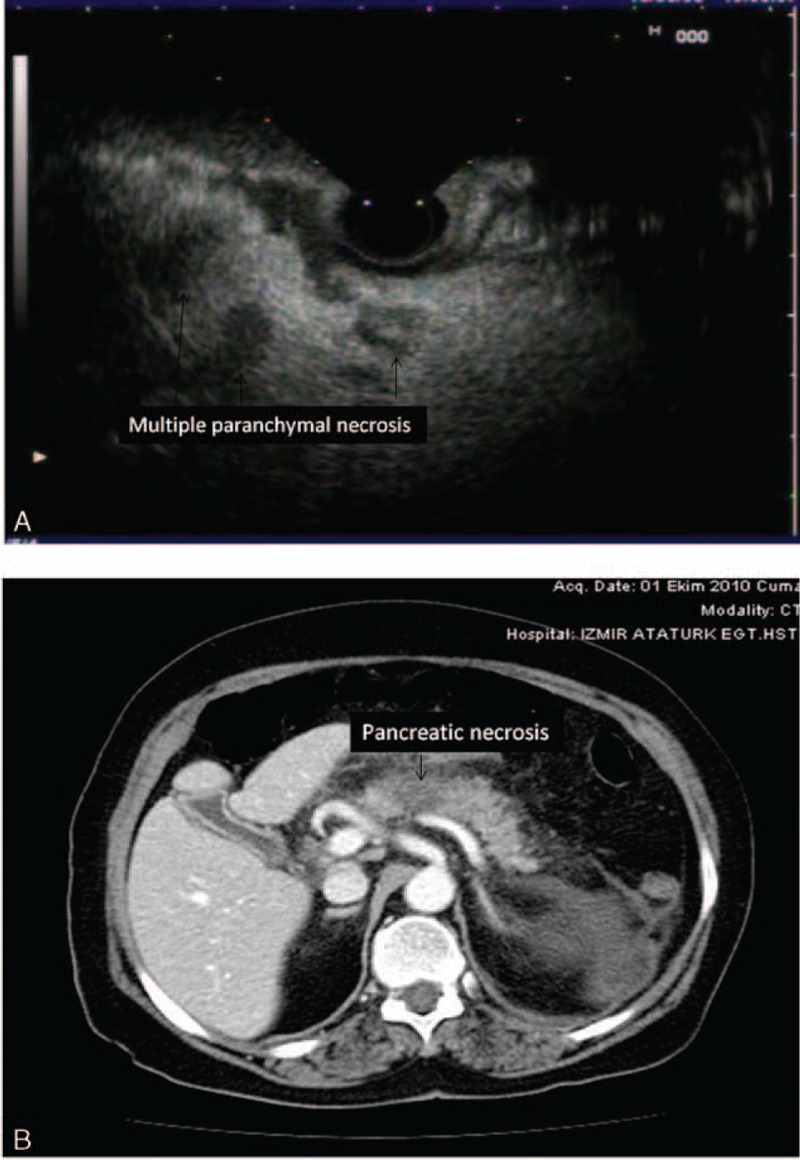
(A) Multiple pancreatic parenchymal necrosis (<20 mm) on EUS are seen (arrowhead). (B) CT view of the pancreatic necrosis (<20 mm) in the same patient. CT = computerized tomography, EUS = endosonography.
